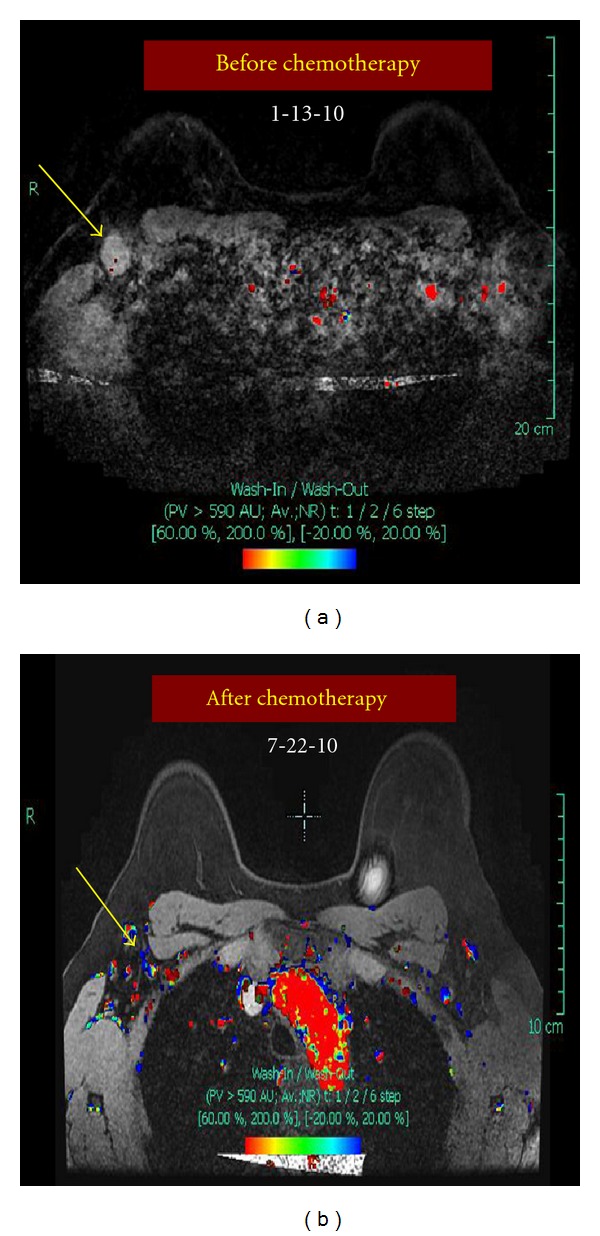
Comparison of bilateral MRI of the right axilla performed before and after neoadjuvant chemotherapy. (a) Doppler flow MRI before neoadjuvant chemotherapy. Yellow arrow points to a 2.6 cm pathologically enlarged right axillary lymph node. (b) Doppler flow MRI after neoadjuvant chemotherapy shows complete radiologic response. The yellow arrow points to the normal-appearing right axillary lymph node.
